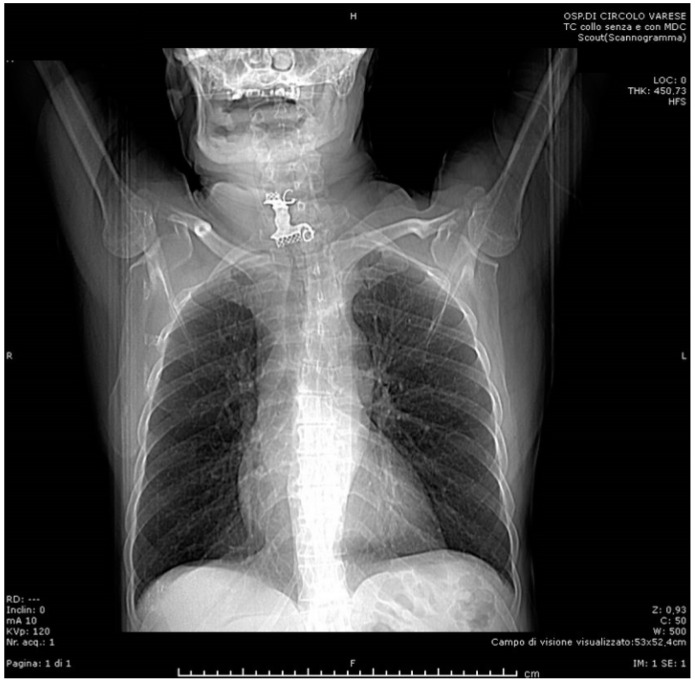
Preoperative CT scan (frontal).
